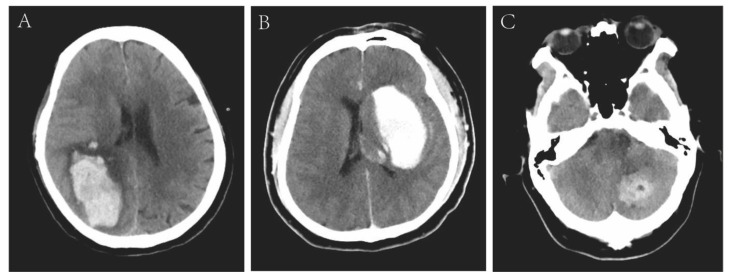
Representative computed tomography images of patients with intracranial hemorrhage. The representative patient (No. 9) had disturbance of consciousness at 72 h after percutaneous coronary intervention (PCI), with bleeding in the frontal, parietal, and temporal lobes (A). Patient (No. 3) had focal neurological dysfunction at 2 h after PCI, with bleeding in the basal ganglia and thalamus (B). Patient (No. 8) had headache and vomiting at 72 h after PCI, and bleeding site was in the left cerebellar hemisphere (C).
